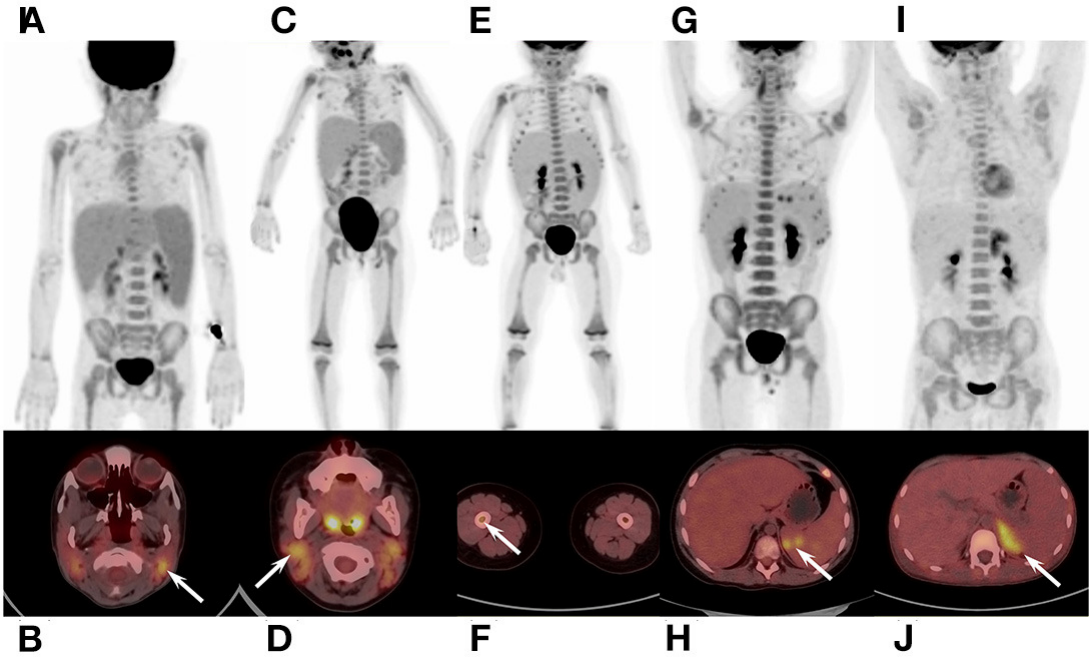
^18^F-FDG PET/CT findings of NM-HLH. (A,B) A 5-year-old girl with EBV-HLH. (C,D) A 3-year-old girl with LPD grade 2. (E,F) a 2-year-old boy with LPD grade 1. (G,H) A 12-year-old boy with LPD grade 2. (I,J) A 11-year-old boy with LPD grade 2. NM-HLH patients usually show multiple lymph nodes with slightly or moderate increased FDG uptake, in bilateral cervical, mediastinal, axillary, retroperitoneal, pelvic and/or inguinal regions (A–D,E,G,J), without extranodal organ involvement (A,C). Sometimes, however, NM-HLH could show extranodal lesions in single organ, such as bone marrow (F), spleen (H), adrenal gland (J), or muscles. HLH, hemophagocytic lymphohistiocytosis; EBV, Epstein-Barr virus; NM-HLH, EBV-induced non-malignancy-associated HLH; EBV-HLH, HLH driven by pure EBV infection; LPD, lymphoproliferative disorders.
